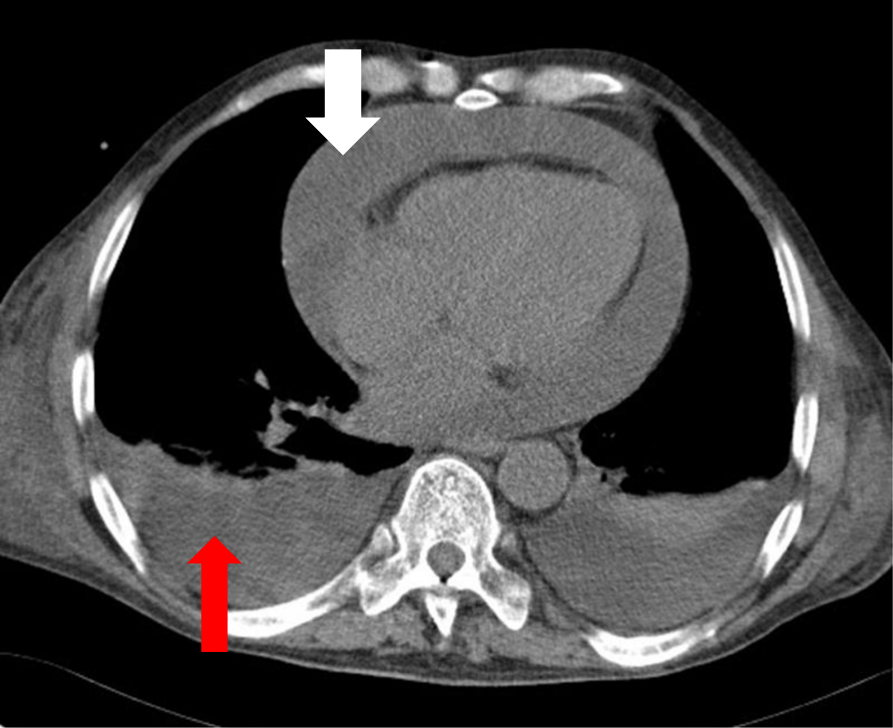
CT of the chest: *white arrow* pericardial effusion, *red arrow* pleural effusion
